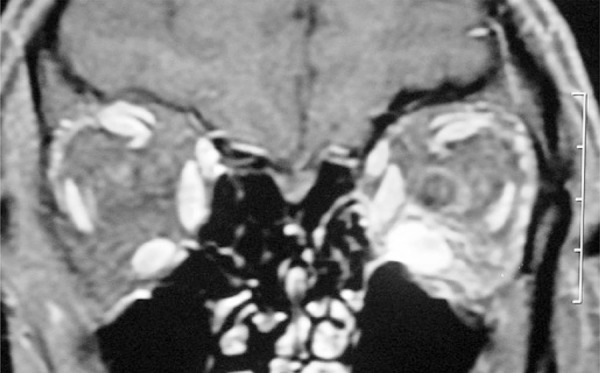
Coronal T1-weighted gadolinium-enhanced magnetic resonance imaging of the orbits showing marked hyperintense enlarged inferior rectus muscle on the left
